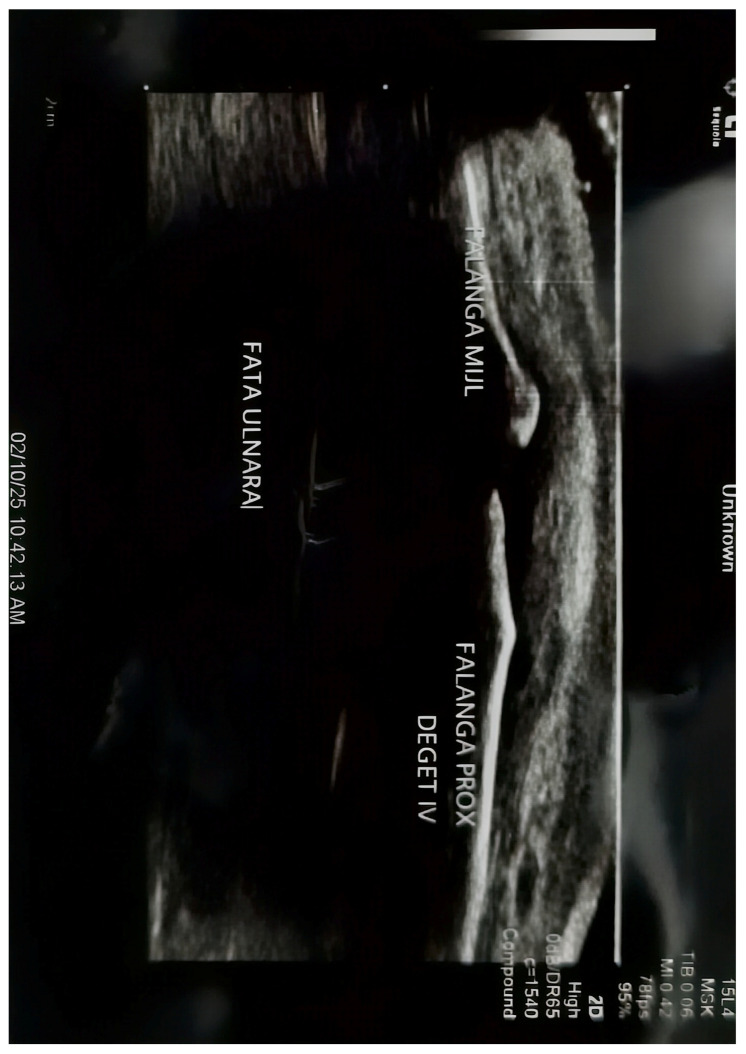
Ultrasound—periarticular soft tissue swelling on the ulnar and radial margins of the PIP joints.
